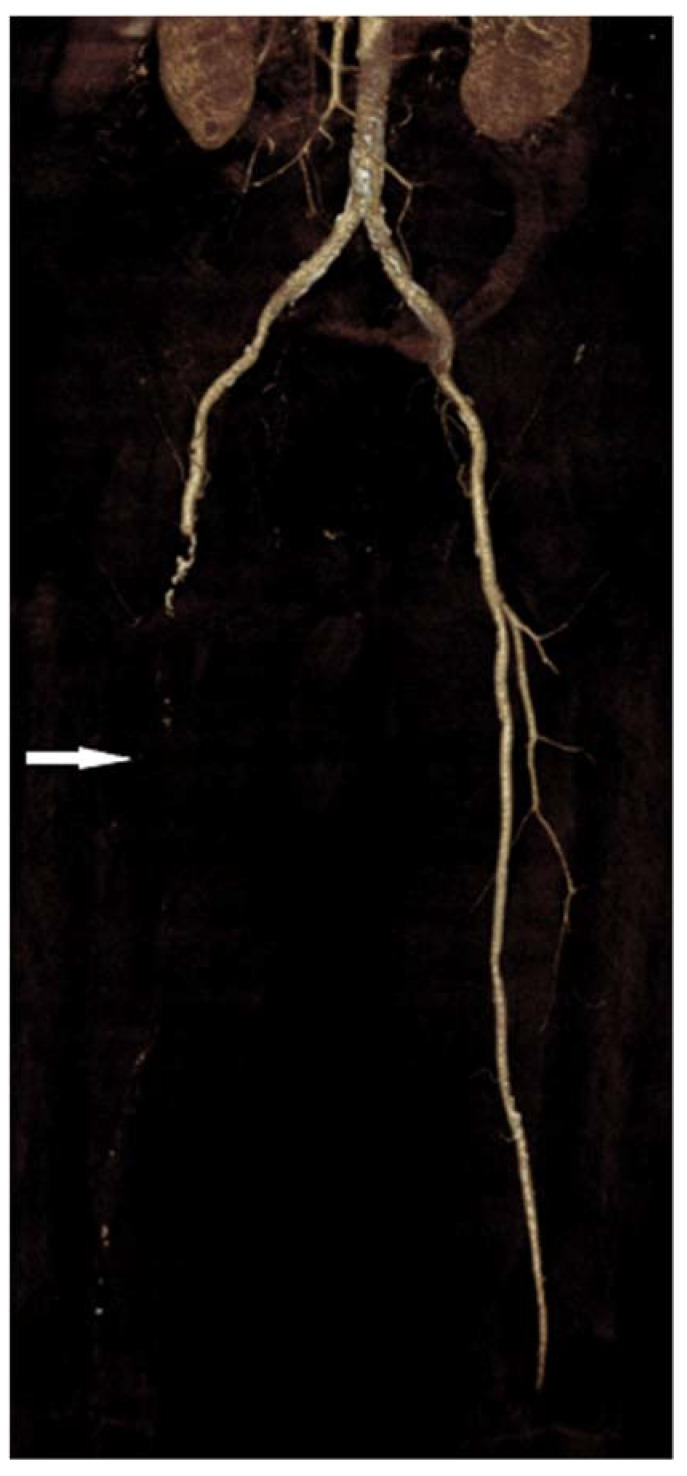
CTA volume-rendered 3D reconstruction from a 66-year-old male demonstrates long segment right superficial femoral artery occlusion (white arrow).
